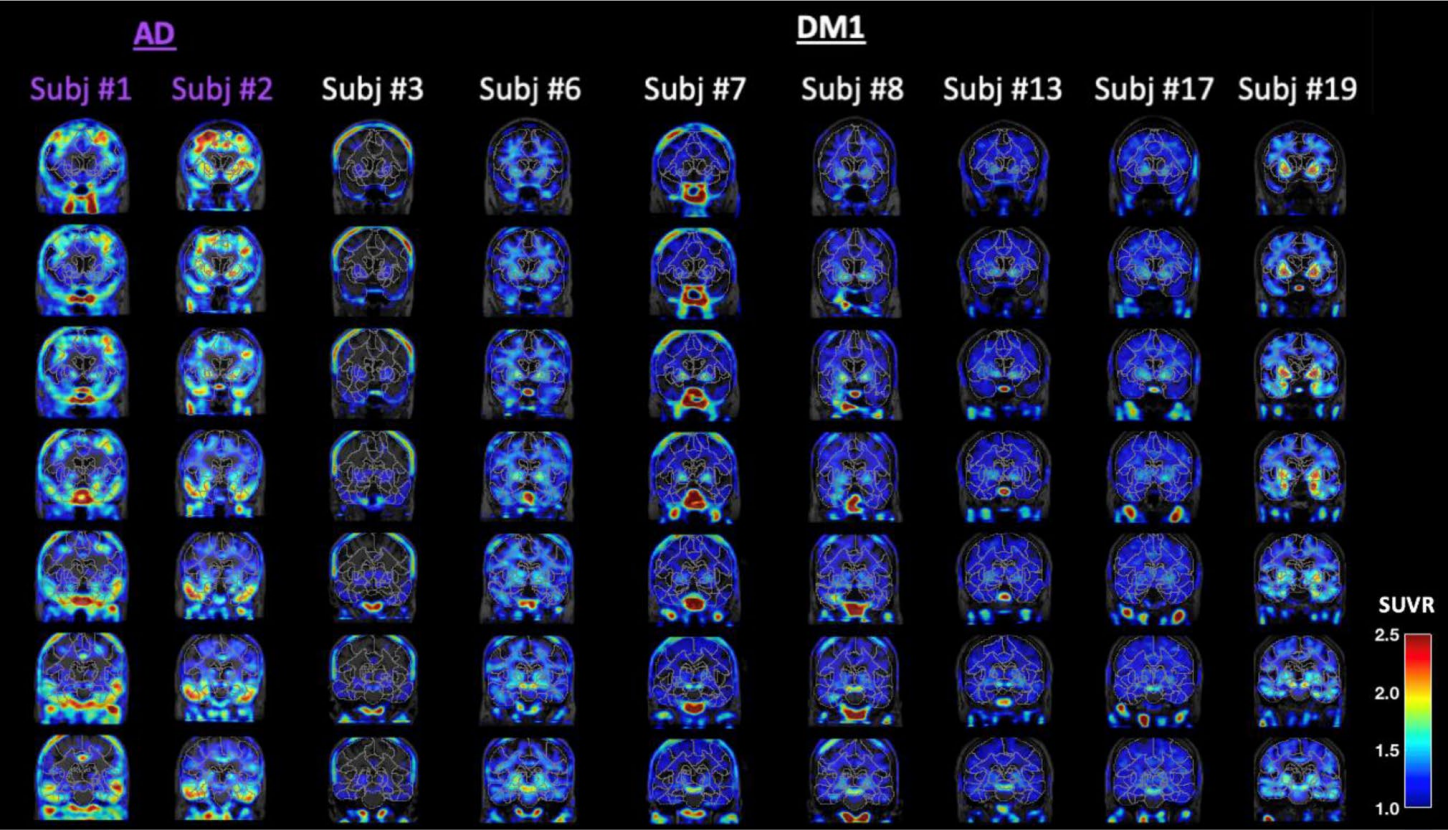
MK-6240 Tau PET images of AD (purple) and DM1 (white) participants. Coronal slices are displayed progressing anterior to posterior. SUVR images are overlaid on subject-specific T1 MRI images, as well as the outline of the subject-specific ROI atlas parcellation. SUVR scale is from 1 to 2.5
